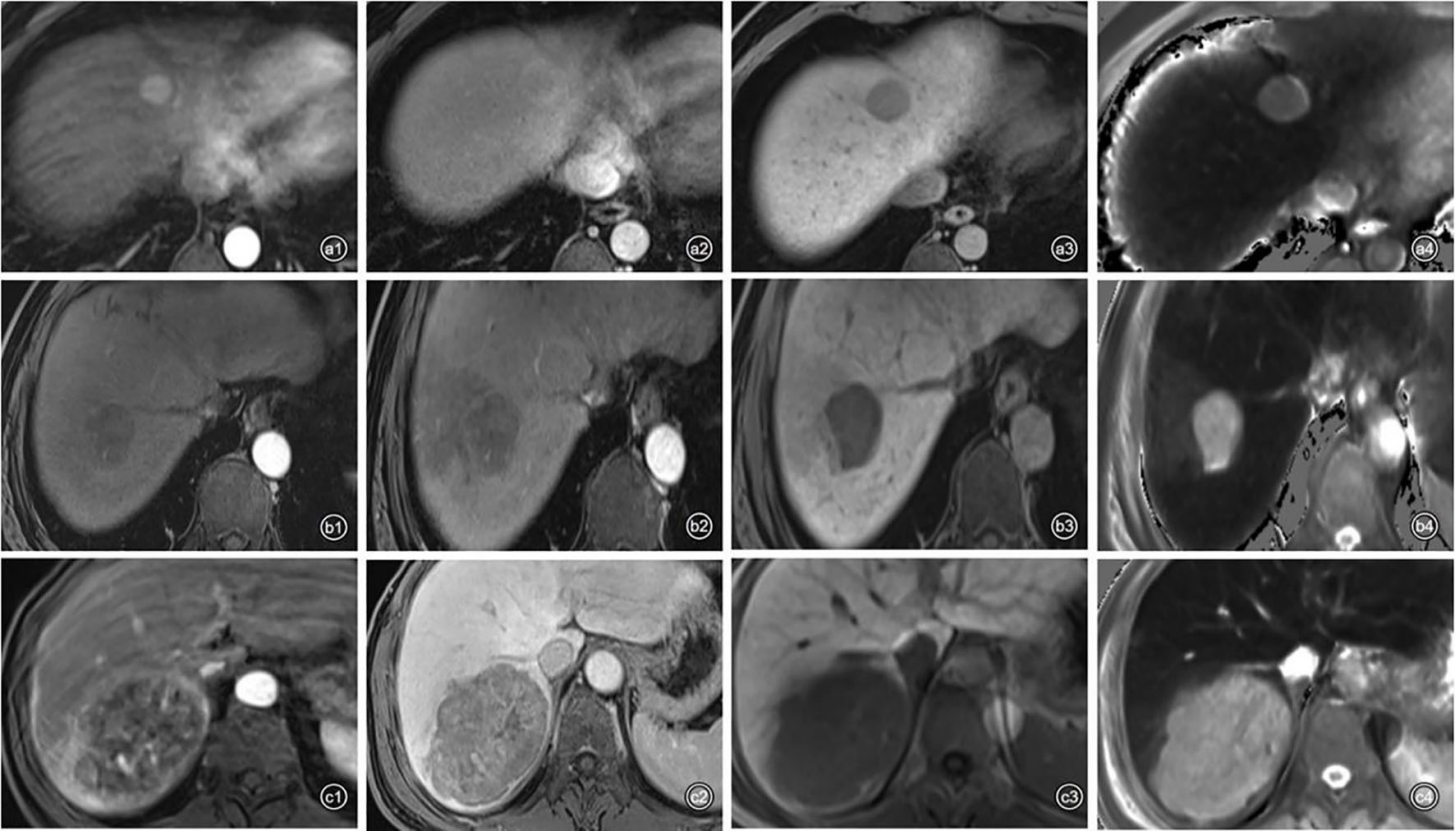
Representative images of each phase on Gd-EOB-DTPA-enhanced MRI and T1 mapping 20 min after enhancement in patients with high or low Ki-67 expression. a1–4: A 48-year-old man with Edmondson–Steiner grade II HCC and low expression of Ki-67 (10%). (a1) APHE; (a2), washout and complete capsule; (a3), smooth tumor margin; (a4), T1rt-20 min = 509.92 ms. b1–4: A 58-year-old man with Edmondson–Steiner grade III HCC and high expression of Ki-67 (30%). (b1) no APHE; (b2), peritumoral enhancement; (b3), peritumoral hypointensity and non-smooth tumor margin; (b4), T1rt-20 min = 1,062.86 ms. c1–4: A 59-year-old man with Edmondson–Steiner grade II HCC and high expression of Ki-67 (40%). (c1), rim enhancement and peritumoral enhancement; (c2), washout; (c3), non-smooth tumor margin; (c4), T1rt-20min = 971.84 ms.
